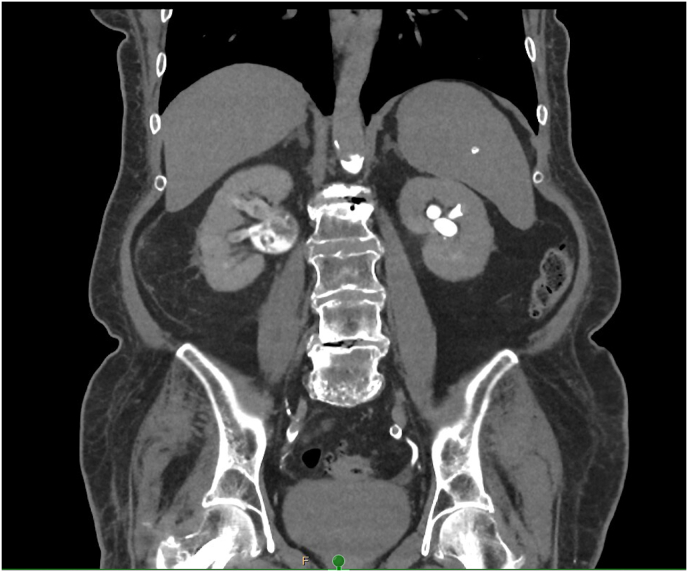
Coronal delayed-phase CT image (30 minutes post-contrast) demonstrating pooling of contrast in the right pyelocaliceal system without contrast passage into the ureter, consistent with complete obstruction at the UPJ.
